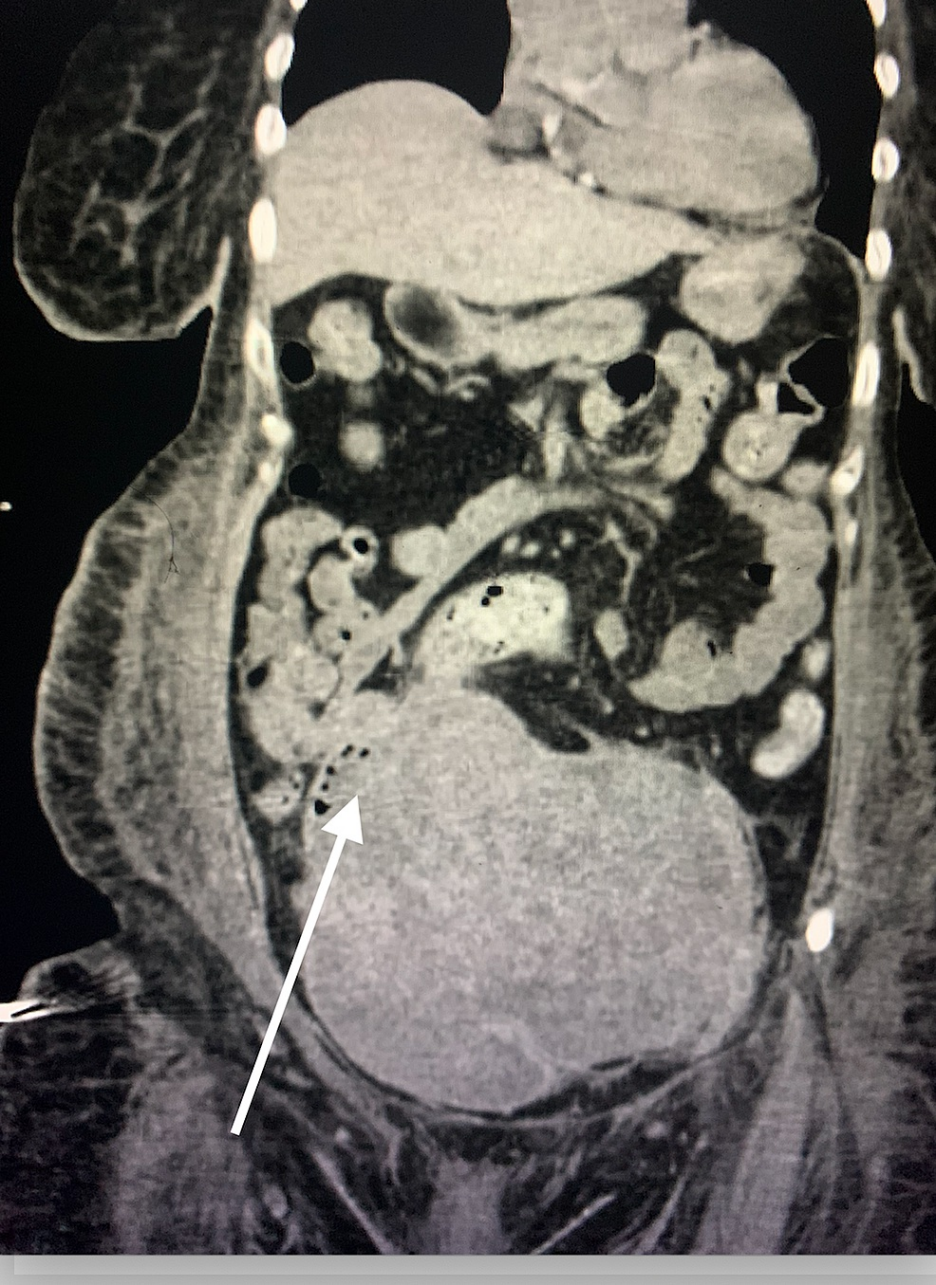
CT scan of abdomen and pelvis showed a large uterine mass with necrotic appearance (white arrow) CT: computed tomography
